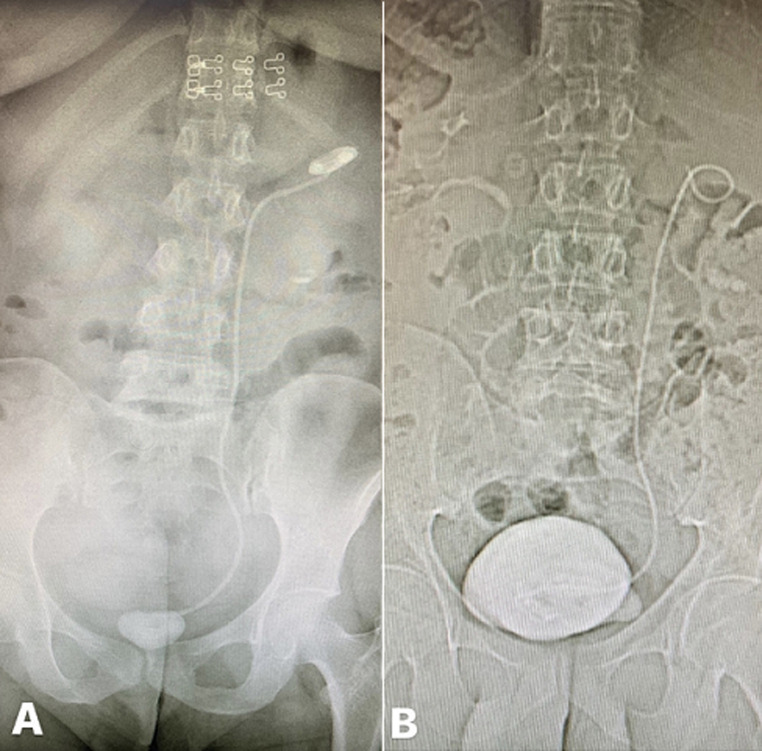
A) plain radiograph showing a retained ureteral stent with encrustation of upper and lower coils; the patient underwent cystolithotrity and percutaneous nephrolithotomy; B) a plain radiograph showing a large bladder stone encompassing the distal coil of a retained ureteral stent
